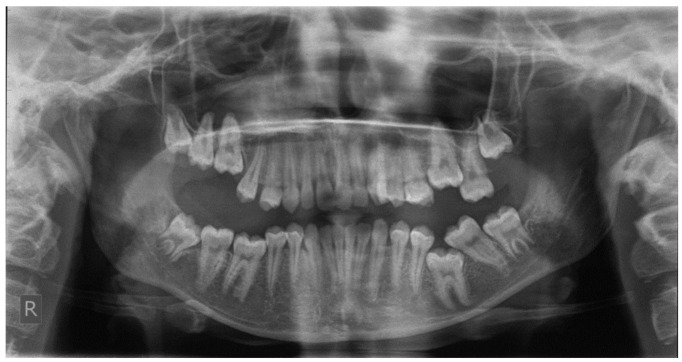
Patient’s orthopantomography presenting Primary Failure of Eruption in all four dental quadrants.
